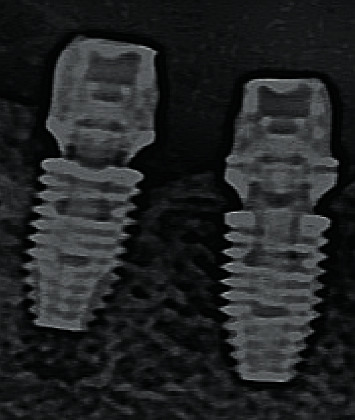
Radiographic control of the fit of the On1 base into the implant during surgery.
